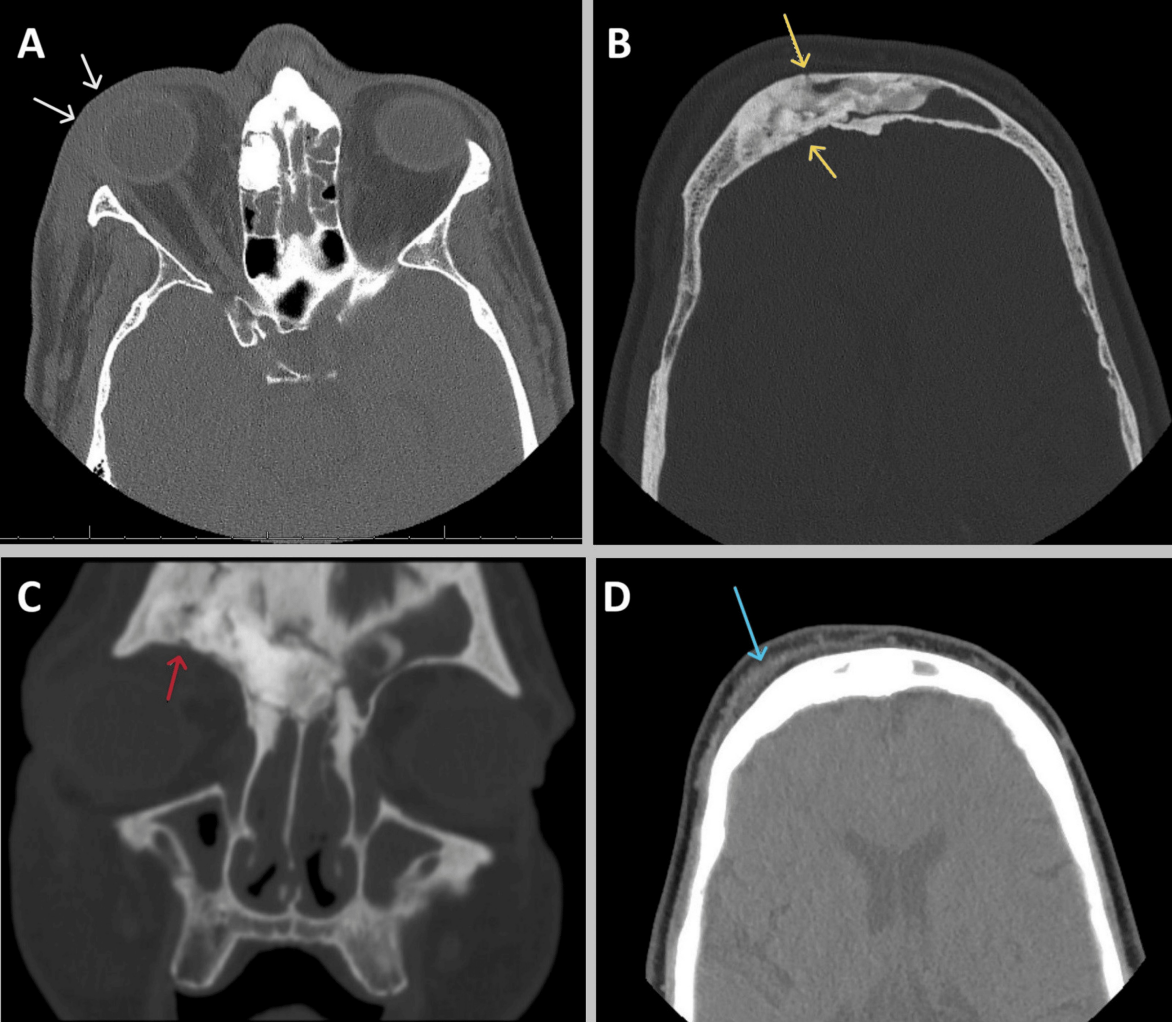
CT of the orbits without IV contrast CT of the orbits without IV contrast showed post-septal fat stranding and edema involving the superior extraconal aspect of the orbit (A: white arrows) compatible with right orbital/post-septal cellulitis and opacification of the frontal sinuses (B: yellow arrows) consistent with severe paranasal sinus disease. There were subtle erosive changes of the right frontal sinus anterior and posterior walls, as well as the floor of the right frontal sinus (right orbital roof; C: red arrow), with possible frontal subgaleal collection (D: blue arrow) secondary to sinus infection.
